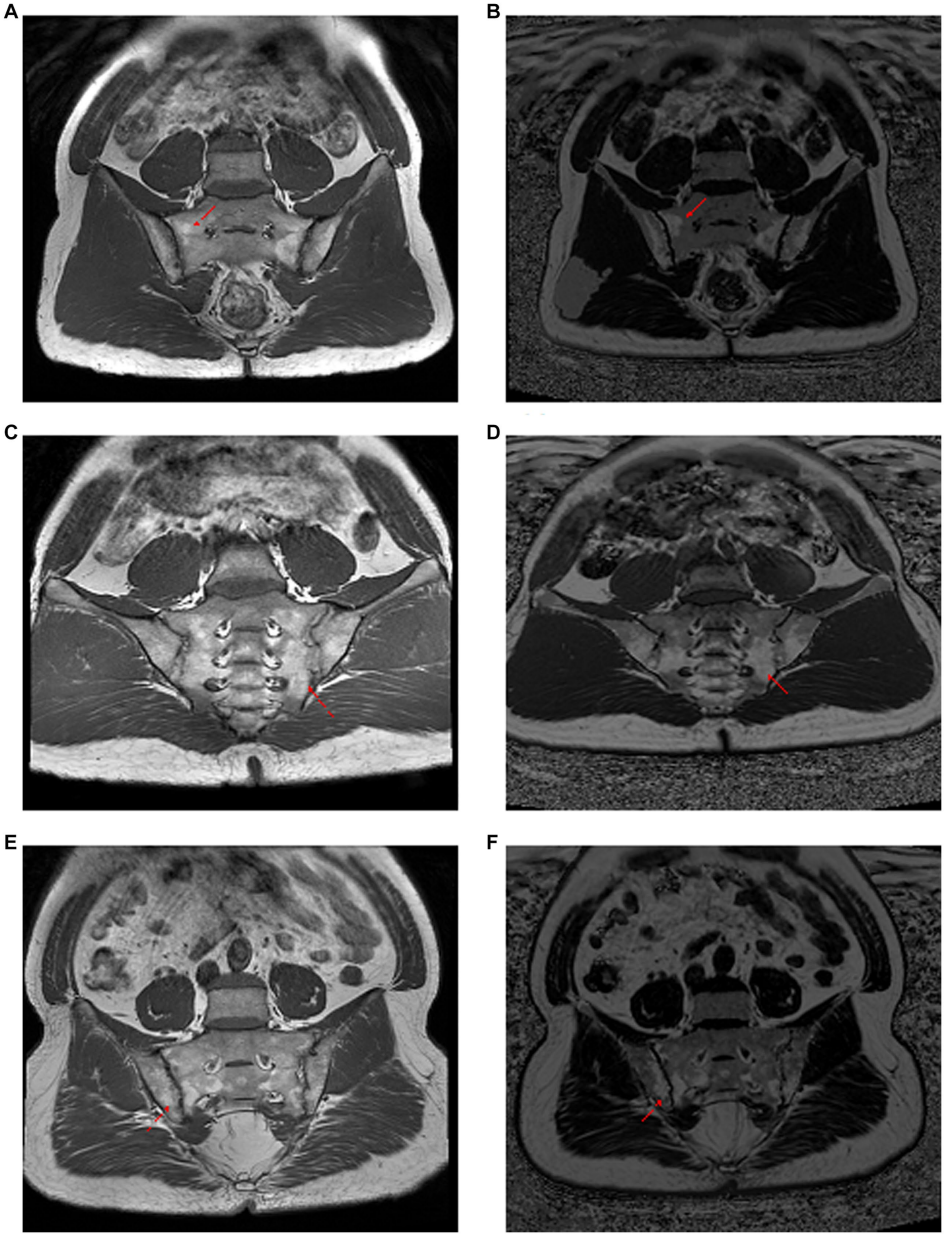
Comparison of T1-weighted images with CSE-MRI fat fraction maps in the observation of fat metaplasia. Panels (A–F) were three different ax-SpA patients. Panels (A,C,E) are T1-weighted imaging images, and panels (B,D,F) are CSE-MRI fat fraction maps. In first and the third patients, the fat deposition pointed by the red arrows are shown as heterogeneous and unclear boundaries on the T1-weighted image, while they are homogeneous and clearly bounded on the CSE-MRI fat fraction map. The second patient had the opposite result. Red arrow: foci of fat deposition.
